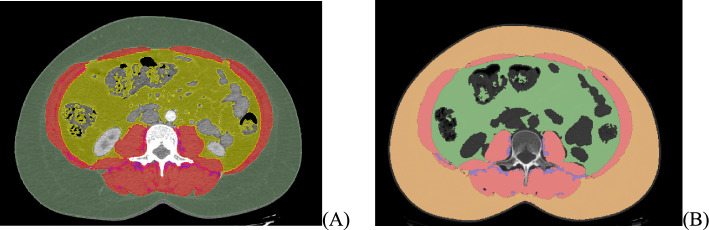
Example of body composition segmentation on the same axial L3 CT slice of a single participant. A Manual segmentation performed in 3D Slicer. B Automated segmentation performed using the IACC software
